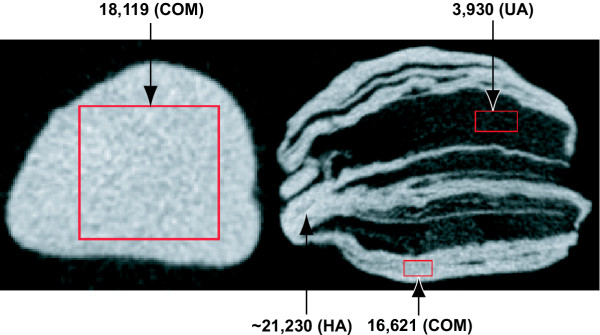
Example of micro CT identification of mineral composition. Visually, there appears to be only one mineral contained in the stone in the left panel and three different minerals that comprise the stone in the right panel. Micro CT attenuation identified the left stone as pure COM, and the right stone as a mixture of hydroxyapatite (bright white, highest attenuation), COM (gray) and uric acid (close to black). Speckled nature of colors – particularly apparent in image at left – is due to image noise as a result of increased magnification.
